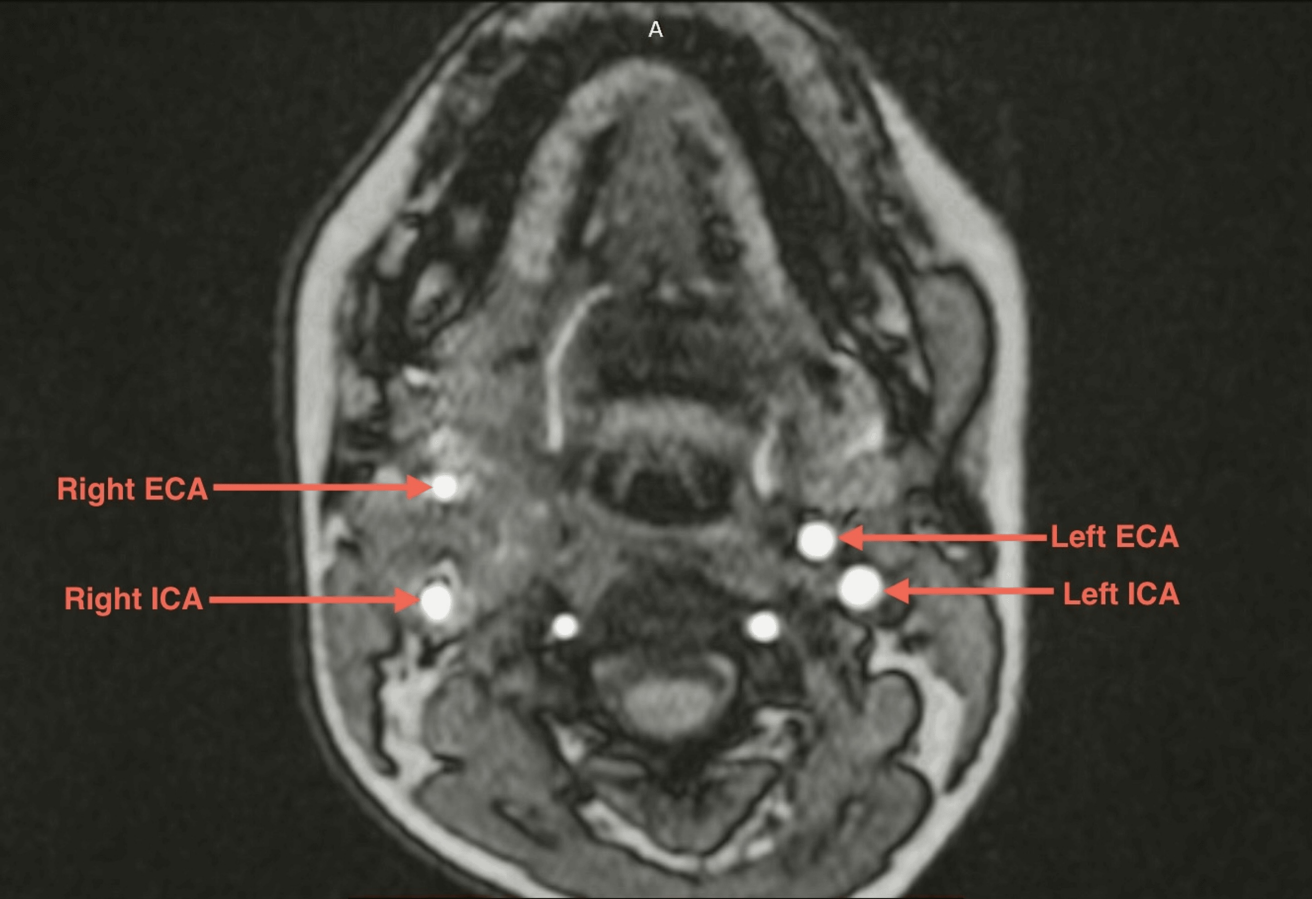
T2-weighted axial section of the magnetic resonance angiography of the head and neck region showing bilateral carotid vessels. Note the difference in the diameter of the right and left vessels on angiogram. ECA: External carotid artery; ICA: Internal carotid artery
